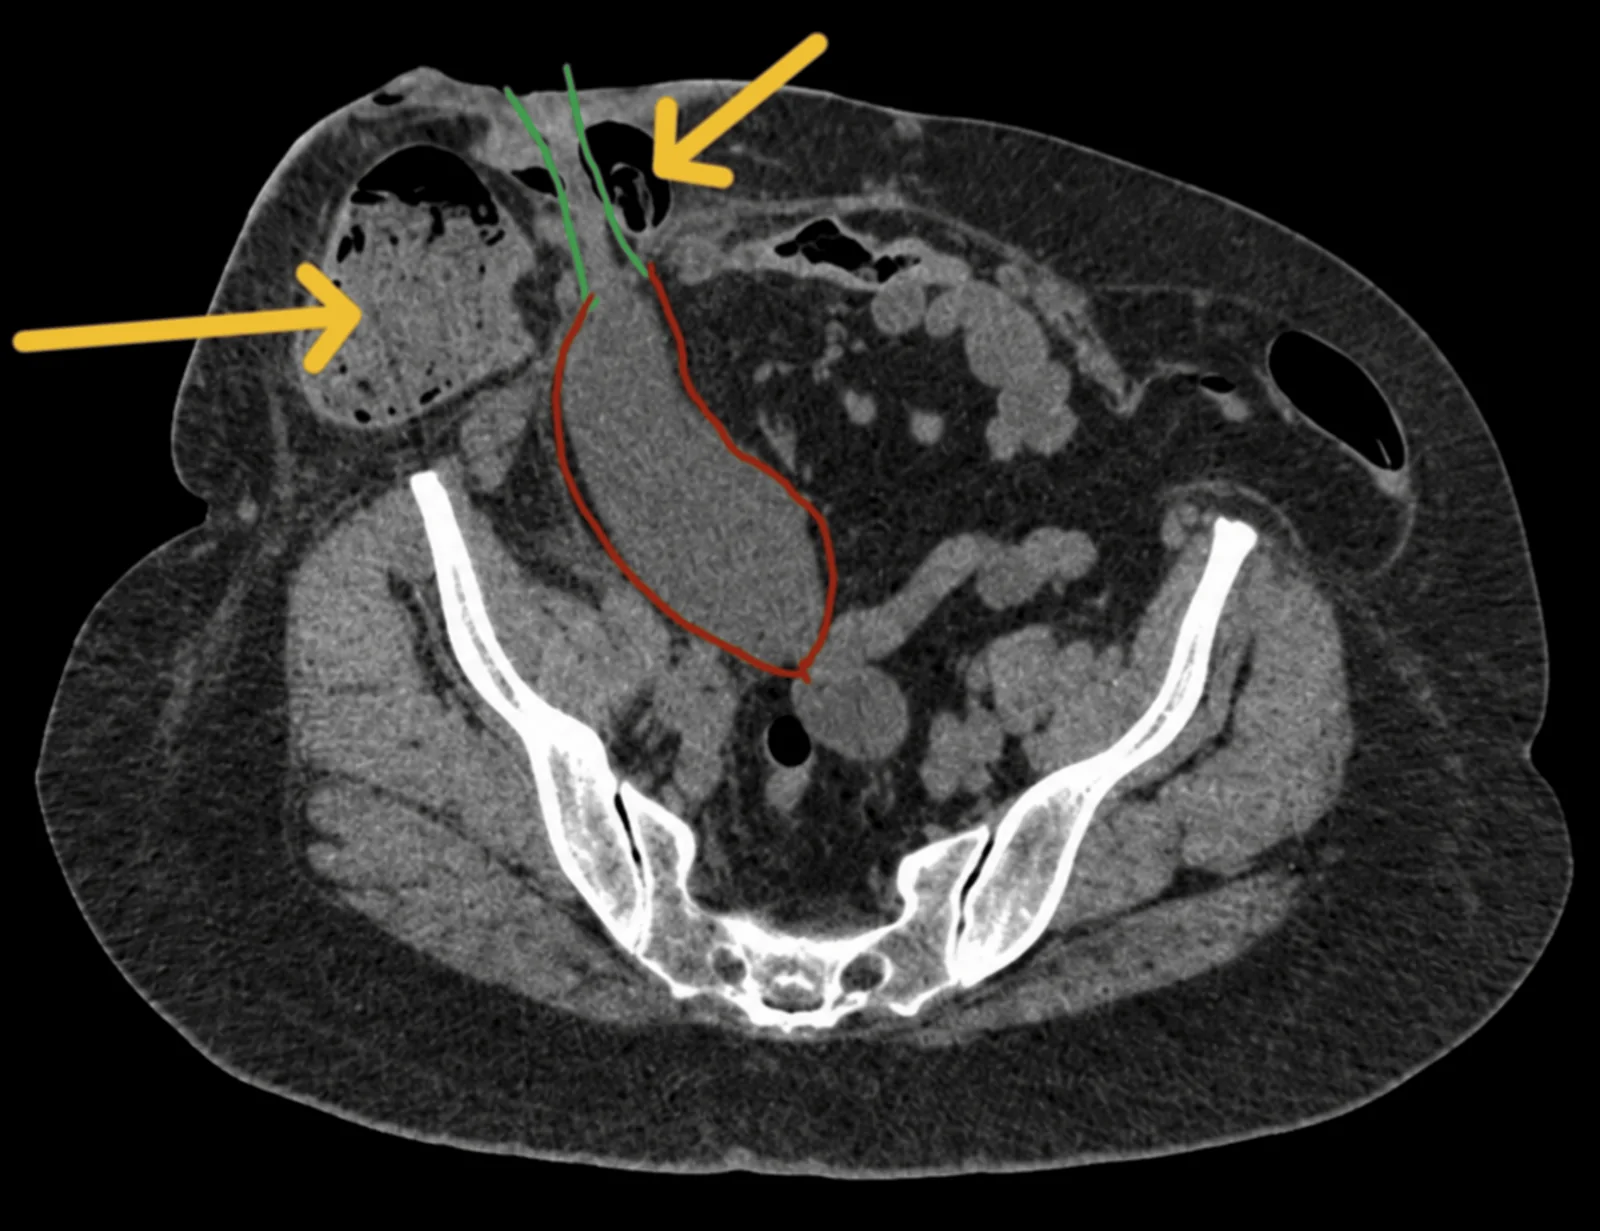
Axial view of CT abdomen The narrow tubular structure between the green lines is the distal segment of the ileal conduit being compressed by the large parastomal hernia shown by the yellow arrows on both sides of the conduit. The structure represented by the red line is the dilated proximal segment of the ileal conduit secondary to mechanical obstruction CT: computed tomography
